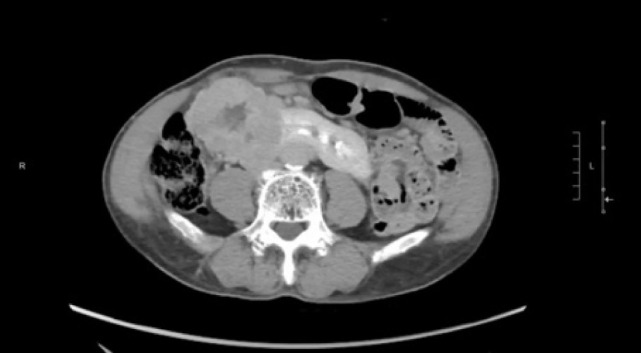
Axial cut of delayed phase-enhanced CT abdomen at the tumor level.
